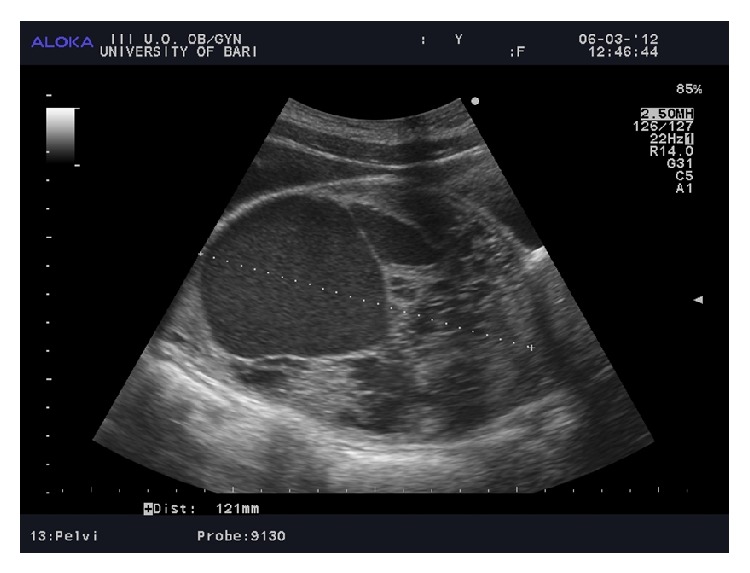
Transabdominal ultrasound showing a right parauterine mass with a complex echogenicity, 12 cm in diameter, deviating the uterus to the left side.
